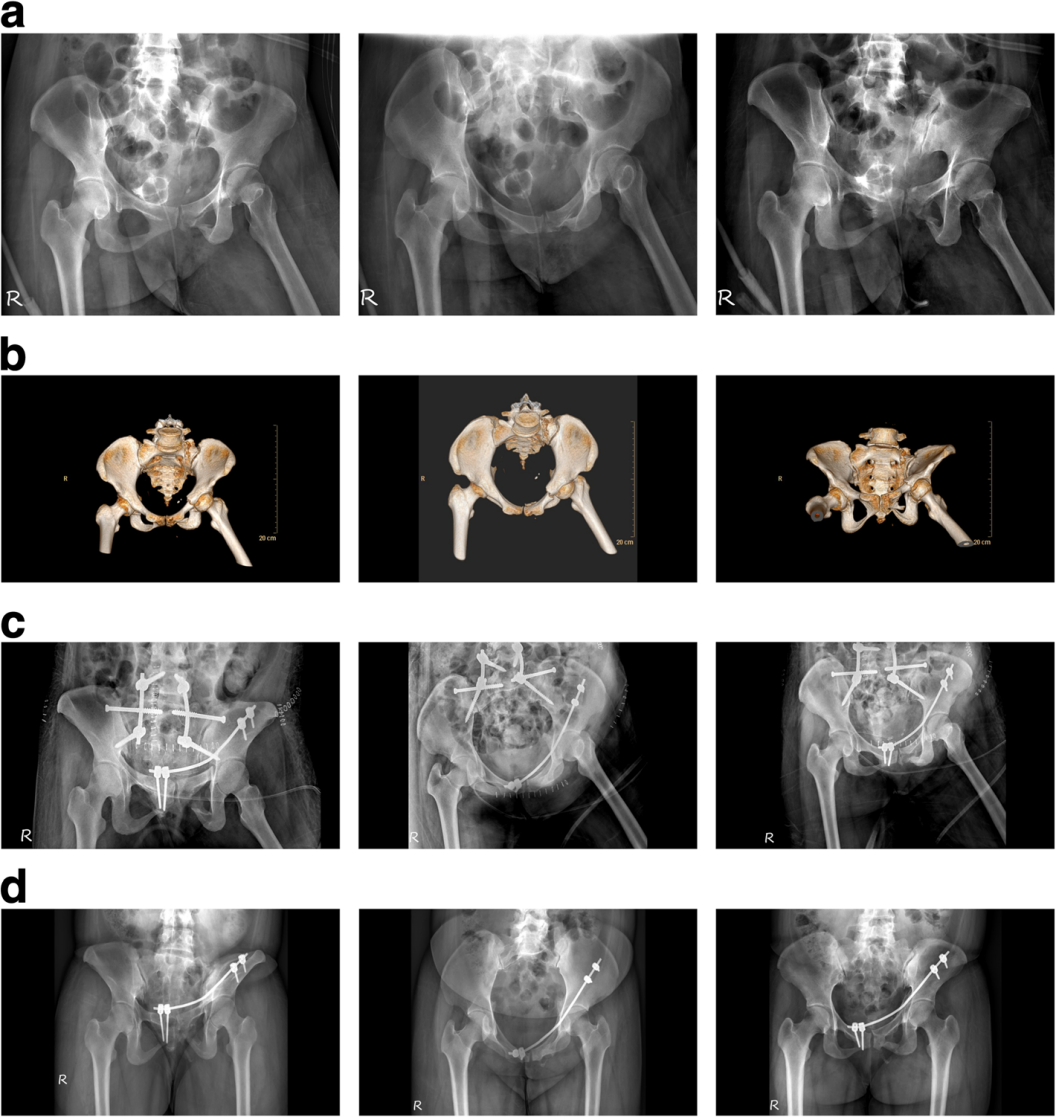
A 39-year-old woman with anterior and posterior pelvic ring injuries caused by a fall. a Preoperative pelvic radiology series (AP, inlet, outlet view) demonstrating left superior and inferior pubic ramus fractures combined with a sacral fracture. b Preoperative 3D reconstructed CT images (AP, inlet, outlet view). c Postoperative pelvic radiology series (AP, inlet, outlet view) demonstrating percutaneous iliosacral screws, spinal pelvic fixation, and the modified pedicle screw-rod fixation. d Postoperative pelvic radiology series (AP, inlet, outlet view) at 22 months follow-up, demonstrating bone union
